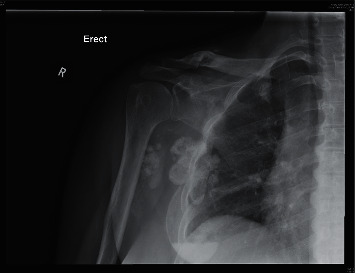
Radiograph of the right shoulder 2 years after teriparatide therapy.
